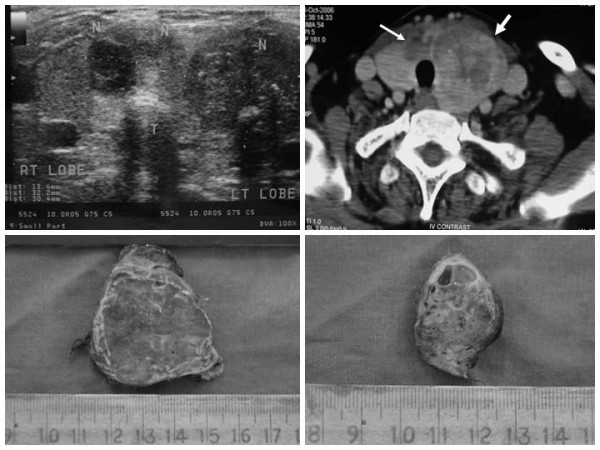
A. USG findings neck. An enlarged heterogenous mass in the left lobe of the thyroid along with another cystic hypoechoic nodule in the isthmus extending into the right lobe (thin arrow). B. CT Scan findings. an enlarged heterogeneous mass with areas of calcification and 'necrosis' in the left lobe of the thyroid (thick arrow) along with another discrete cystic nodule in the isthmus (thin arrow). C. Gross findings. A solid, fleshy, grey white tumor in the left lobe of the thyroid. D. Isthmic nodule exhibiting multiple cystic areas.
